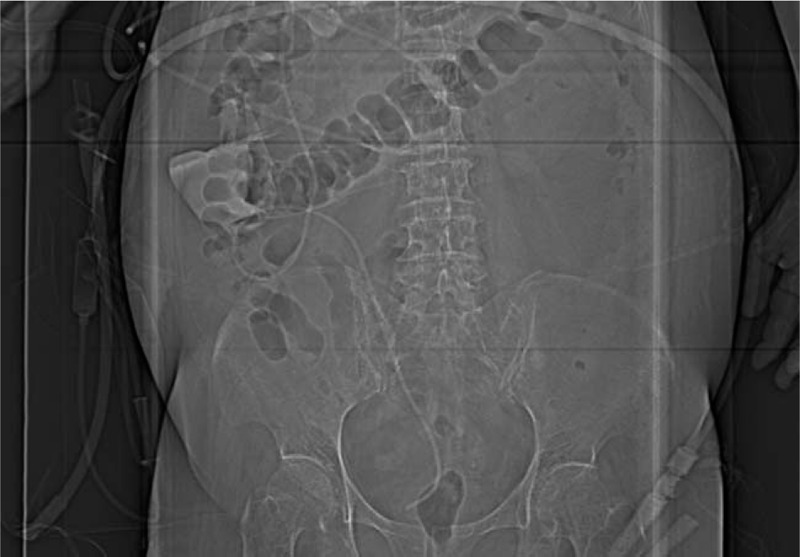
Postoperative head CT scan obtained during the second admission revealed the relief of ventricular enlargement and paraventricular edema. CT = computed tomography.
